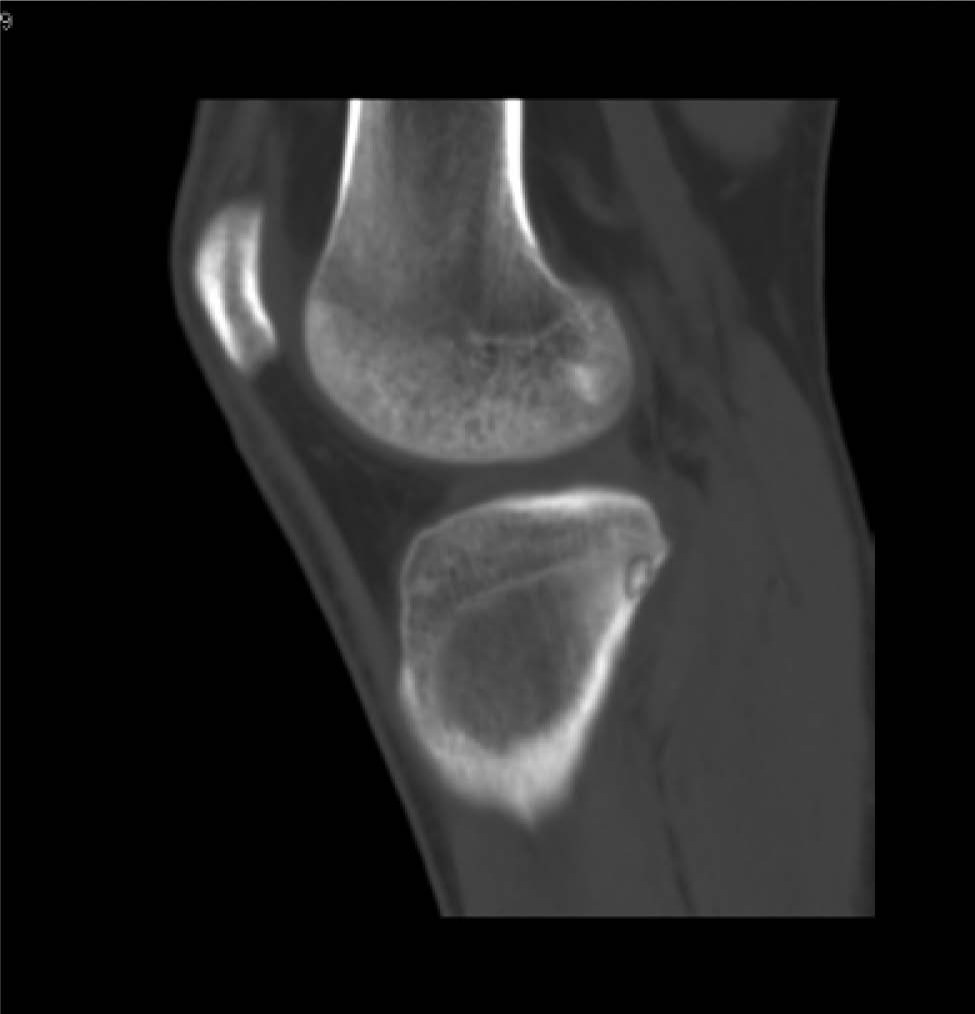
Preoperative CT image in mid-sagittal plane. CT = computed tomography.
